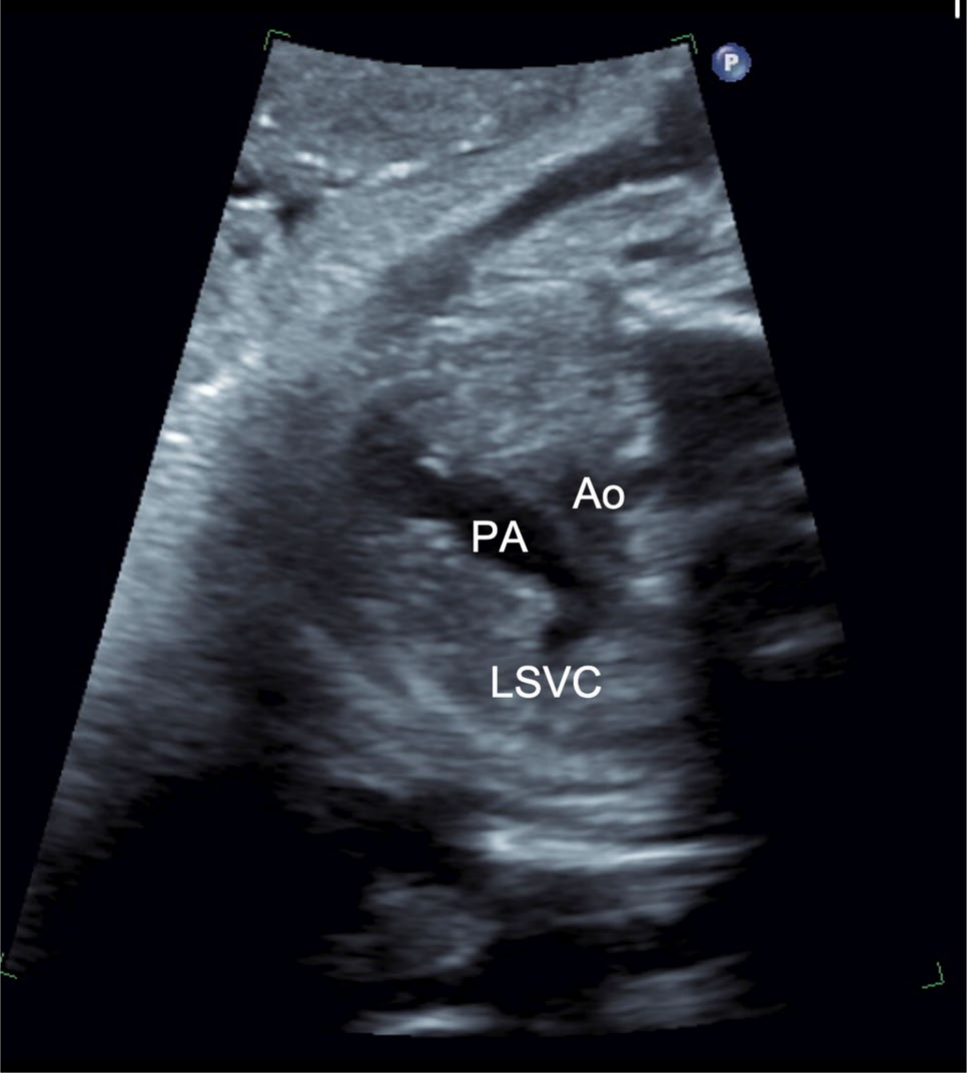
Abnormal three-vessel view on fetal echocardiogram, from left to right showing the PLSVC, Pulmonary Arteries, and Aorta
